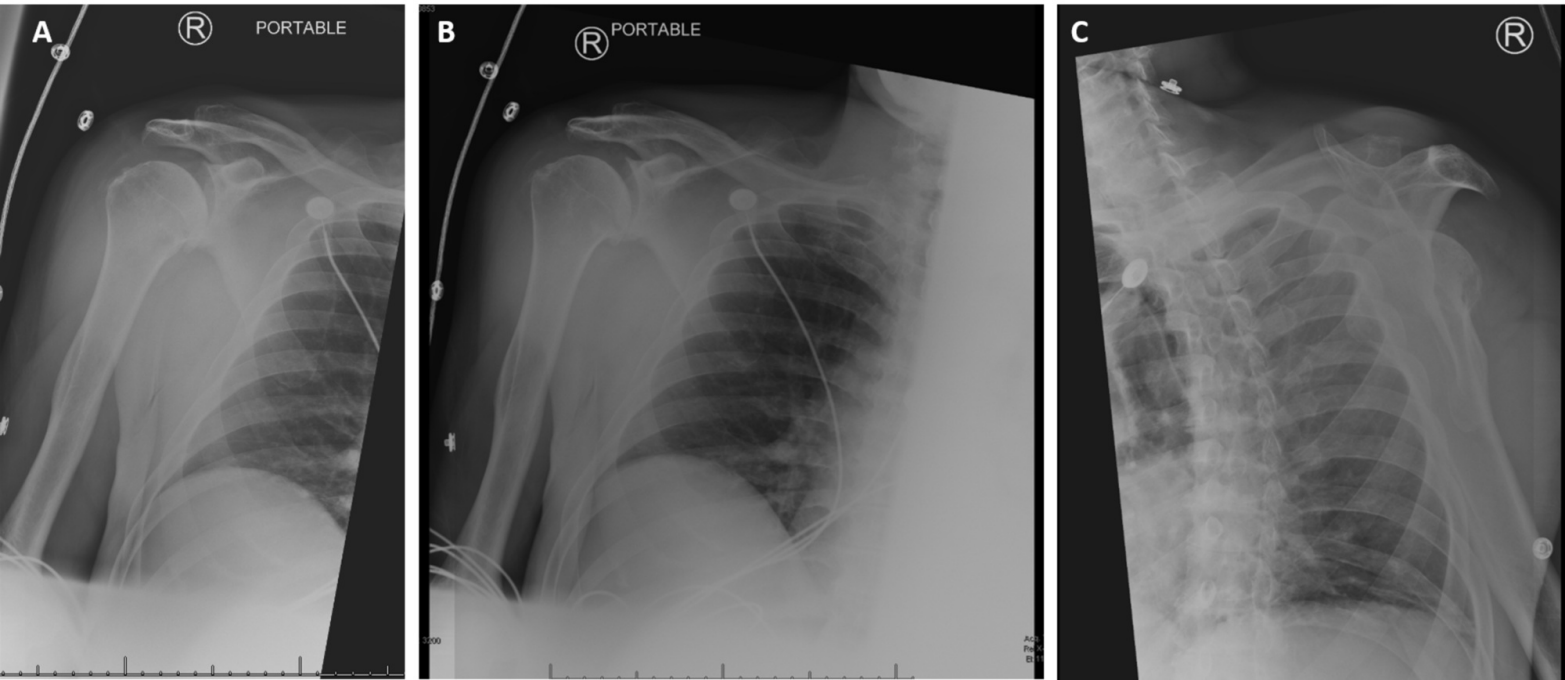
Right shoulder X-ray of the patient
